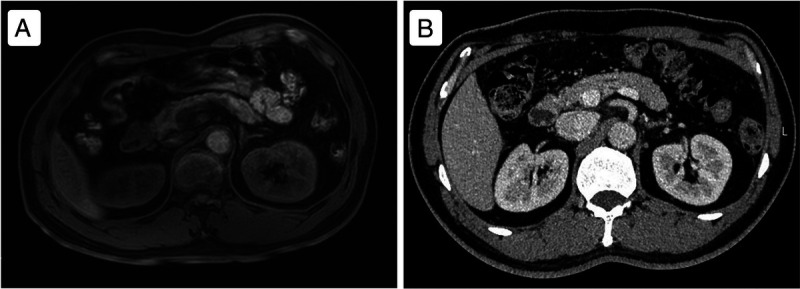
Questionable duodenal lesion. A, Abdominal magnetic resonance imaging revealed duodenal wall thickening, suggestive of angioneurotic edema. B, Enhanced abdominal computed tomography showed the thickening of the duodenal wall and luminal narrowing; the boundary between this affected area and the pancreas was ambiguous.
